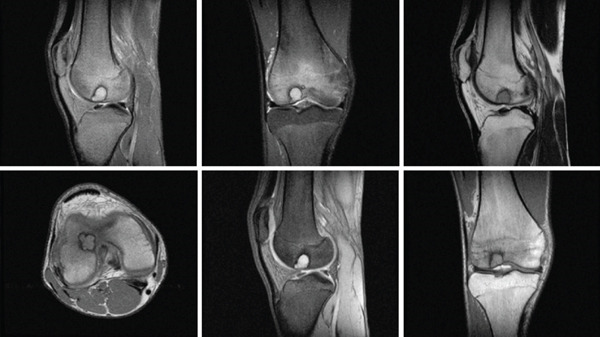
Preoperative T1‐ and T2‐weighted MRI of the right knee revealing bone contusion within the lateral femoral condyle, without any evidence for displacement of fragment or collapse.
